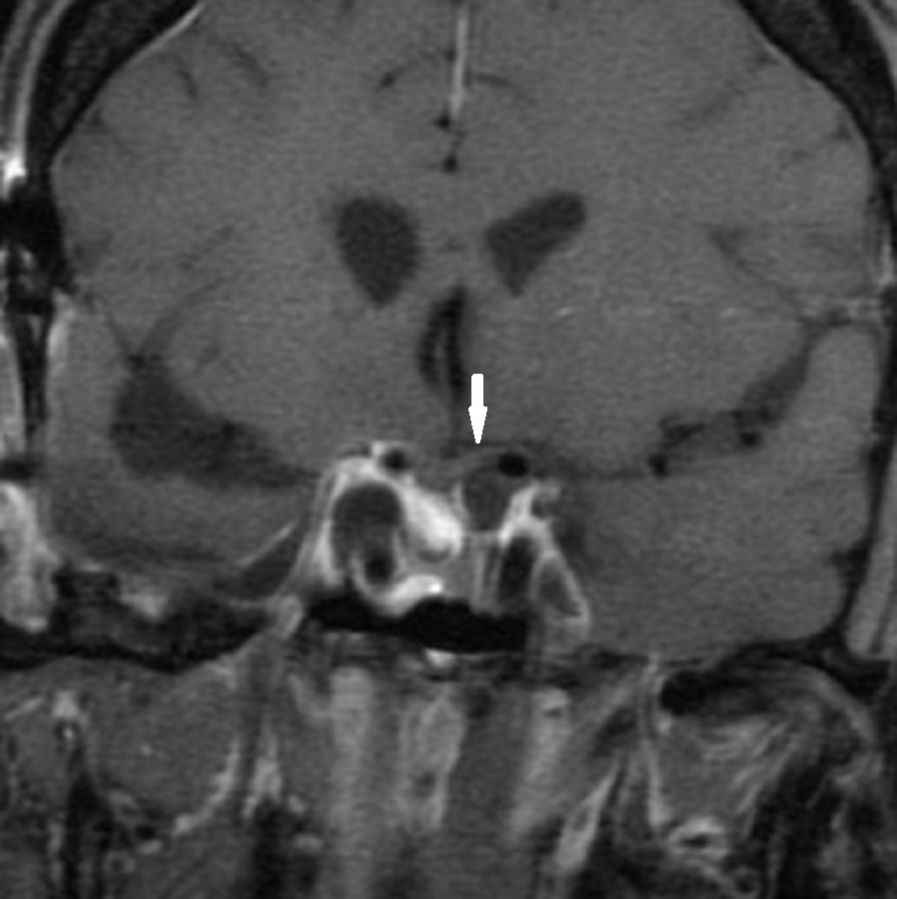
Coronal Post-Contrast MRI Sequences Sequential tumor regression over eight cycles of TMZ treatment. Note the prominent reduction in tumor volume and gadolinium enhancement.
